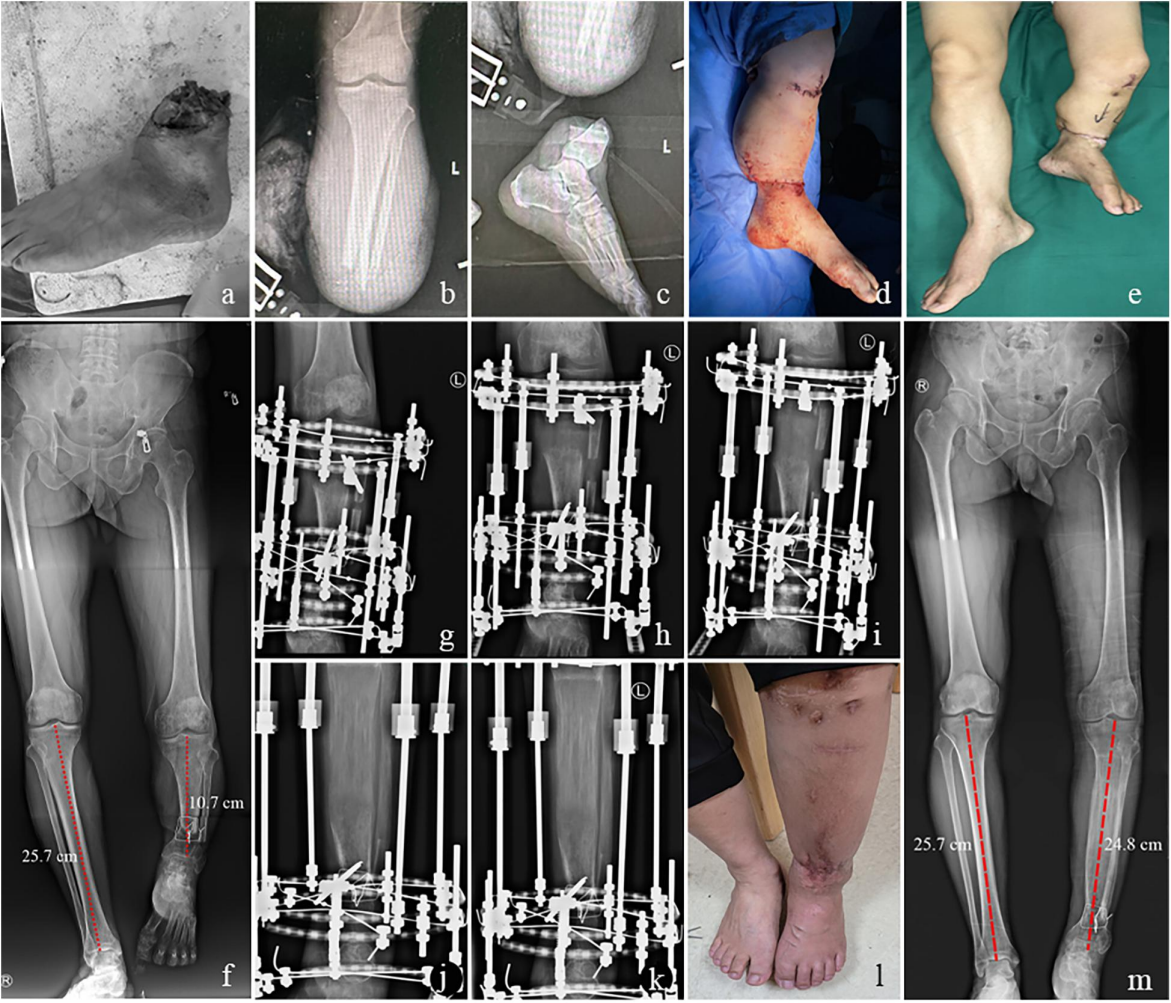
A 51-year-old male with limb shortening deformity after replantation of lower leg amputation treated by limb lengthening using an external fixator. (a) Traumatic amputation of the distal left lower leg. (b,c) x-rays of left tibia. (d) Replantation of the affected limb after shortening approximately 15 cm. (e) Successful limb salvage. (f) The full-length x-ray of the lower limb showed a shortening of about 10 cm in the left lower leg. (g) Postoperative x-ray of the left tibia. (h–j) x-ray of the left tibia at one month, 3 months and 5 months after surgery. (k,l) The consolidation phase was completed with satisfactory bone union and limb function recovery at the 21 postoperative month. (m) The full-length x-ray of the lower limb revealed a shortening of approximately 0.9 cm in the left lower leg, which is generally considered acceptable.
